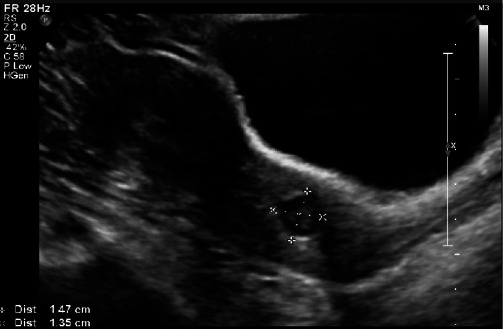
Transabdominal and sagittal ultrasound 12 weeks after removal of balloon demonstrating GS decreased to 1.4 cm.
